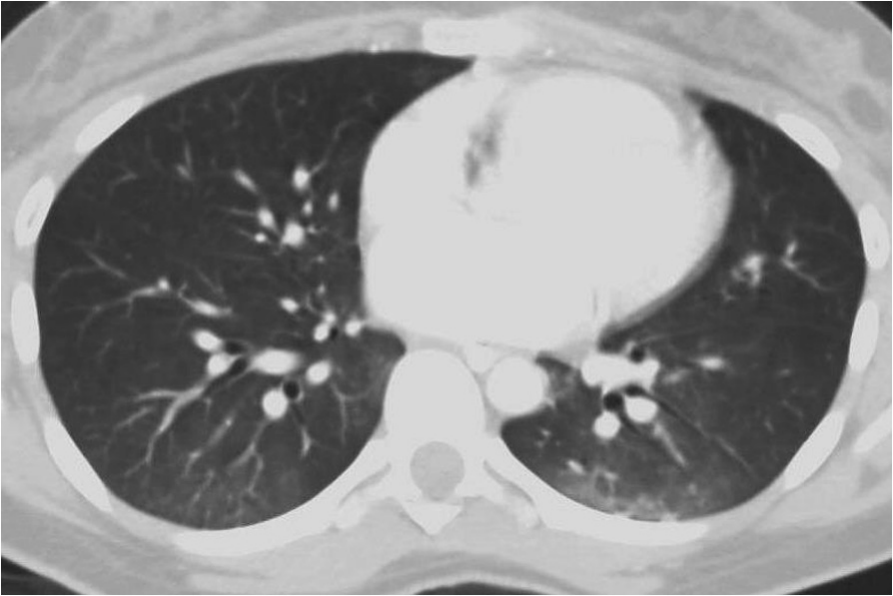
Admission CT scan of 18 year-old female with severe TBI (no pulmonary injuries identified).
